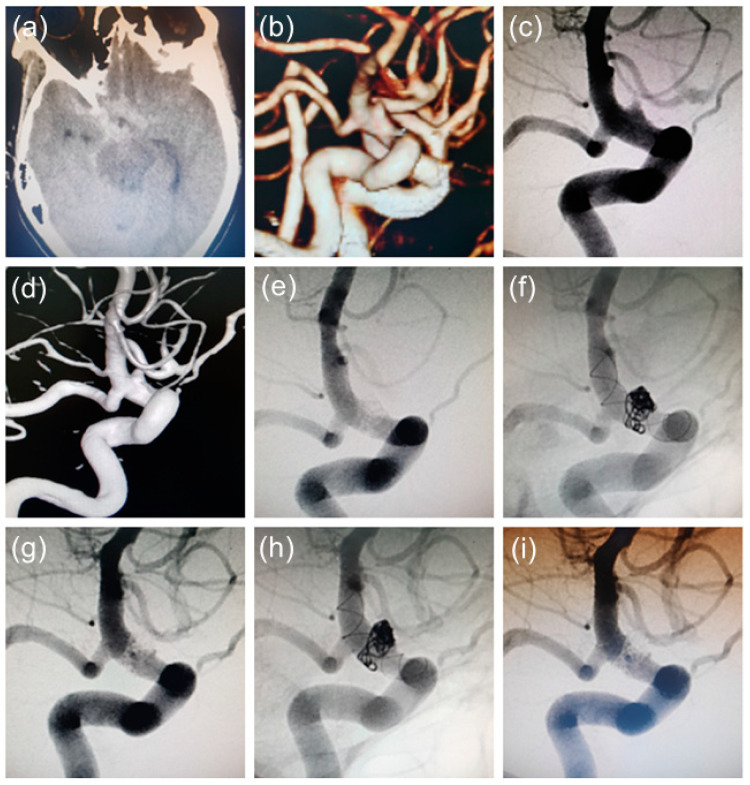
53-year-old female presented with severe headache accompanied by unconsciousness, and an axial CT head showed a subarachnoid hemorrhage (a). A pretreatment CT angiogram exhibited an aneurysm in the clinoid segment of the right intracranial artery (b). DSA examination and 3D reconstruction cleared the ruptured blood blister-like aneurysm (treatment angle) (c,d). During the process, the patient underwent FDD-assisted coiling via a semi-deploying method (Tubridge 3.5 × 20 mm). After treatment, the aneurysm was completely occluded (e,f). Follow-up angiograms at 3 months (g,h) and 6 months post-procedure showed complete resolution of the aneurysm with remodeling of the parent vessel (i).
